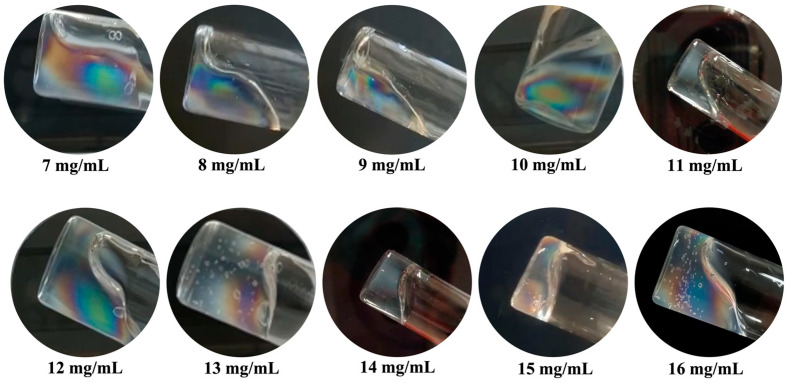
Images of CT-DNA/ TE buffer at C_DNA_ from 7 to 16 mg/mL and stored in vials (inner diameters in the range of 2 cm) observed under gentle flow between crossed polarizers. Observations were carried on at room temperature.
